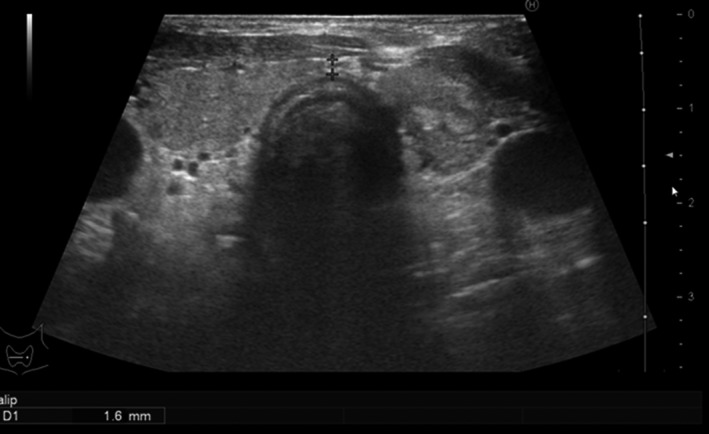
Ultrasound showing that this patient had a normal‐sized thyroid with small nodules on admission.
